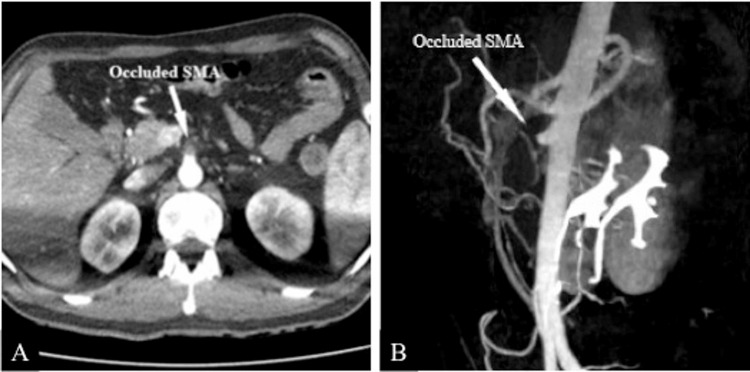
CTA findings of the SMA occlusion. A: Axial view; total occlusion of the SMA; B: Sagittal view; total occlusion of the SMA, no recanalization. SMA: superior mesenteric artery
